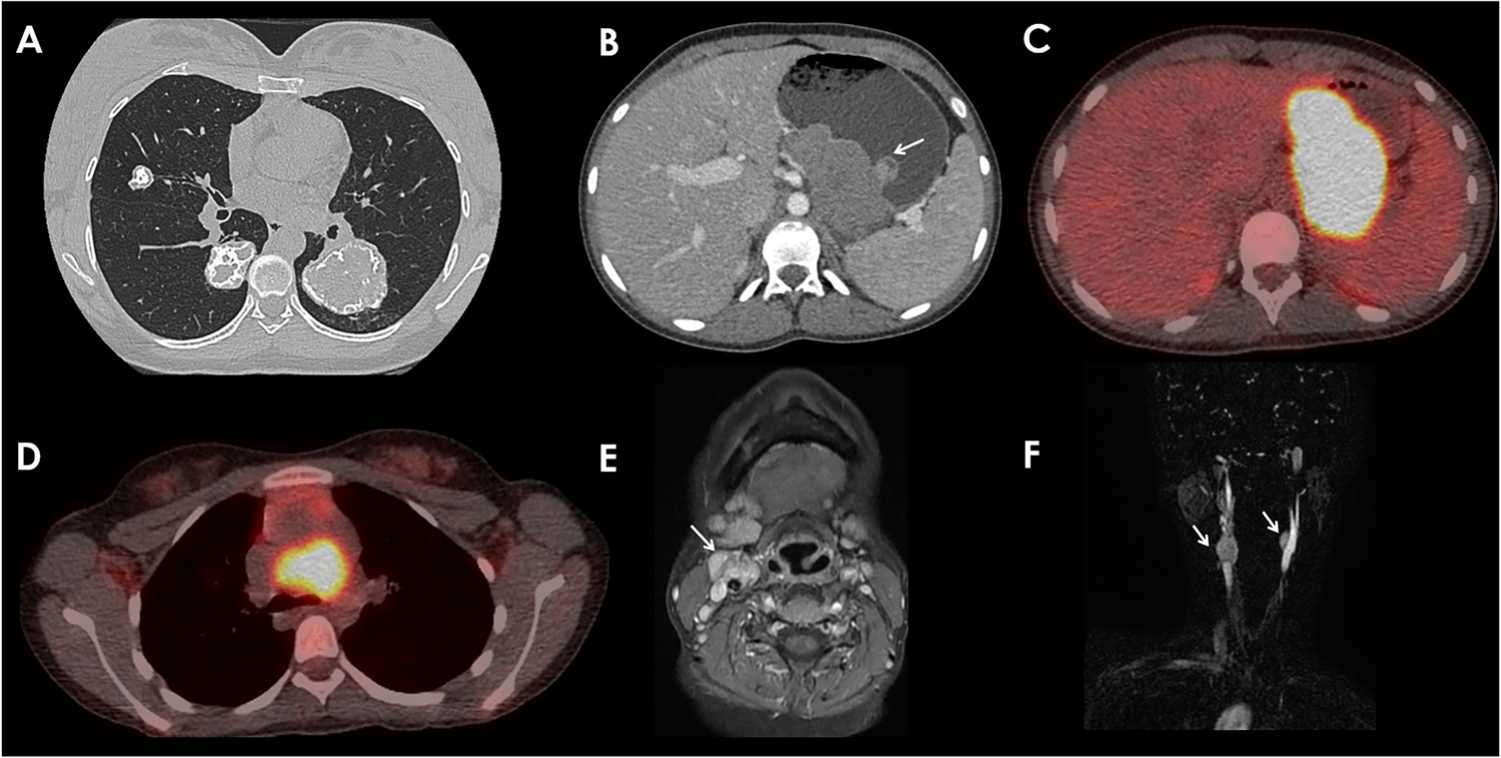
14 y.o. patient with SDHC Mutation presenting Carney Triad, which is a rare association of an extra-adrenal PGL, with pulmonary chondroma and a GIST. A Axial parenchyma CT Scan view of pulmonary chondroma, associated with a heterogeneous lobulated submucosal gastric mass corresponding to a mesenchymal tumors: GIST seen on the portal venous phase CT scan (B), high uptake at ^18^F-FDG PET-CT SUVmax 30.3 (C). In addition, there’s a mediastinal paraganglioma with a high uptake at 18F-FDG PET/CT SUV 10.2 (D), and cervical carotid body PGL: (E) T1-w CE MRI and Time-resolved imaging of contrast kinetics (Tricks®) MRA (F)
